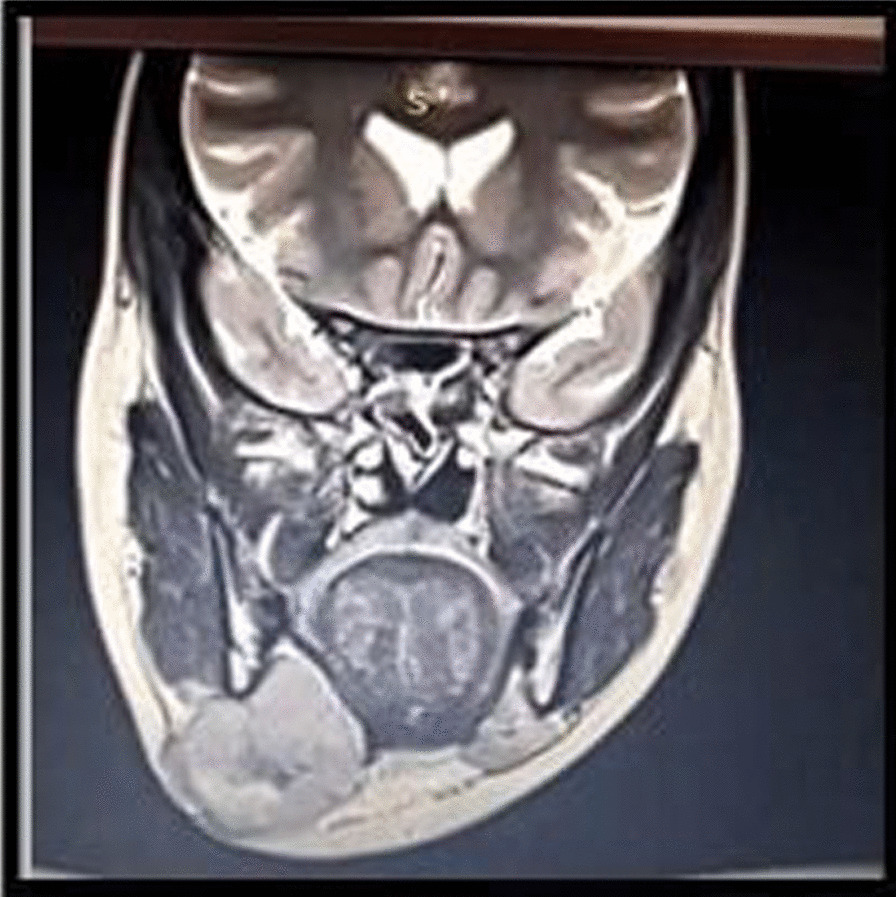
MRI scan showing well defined lobulated mass seen in the right submandibular region
